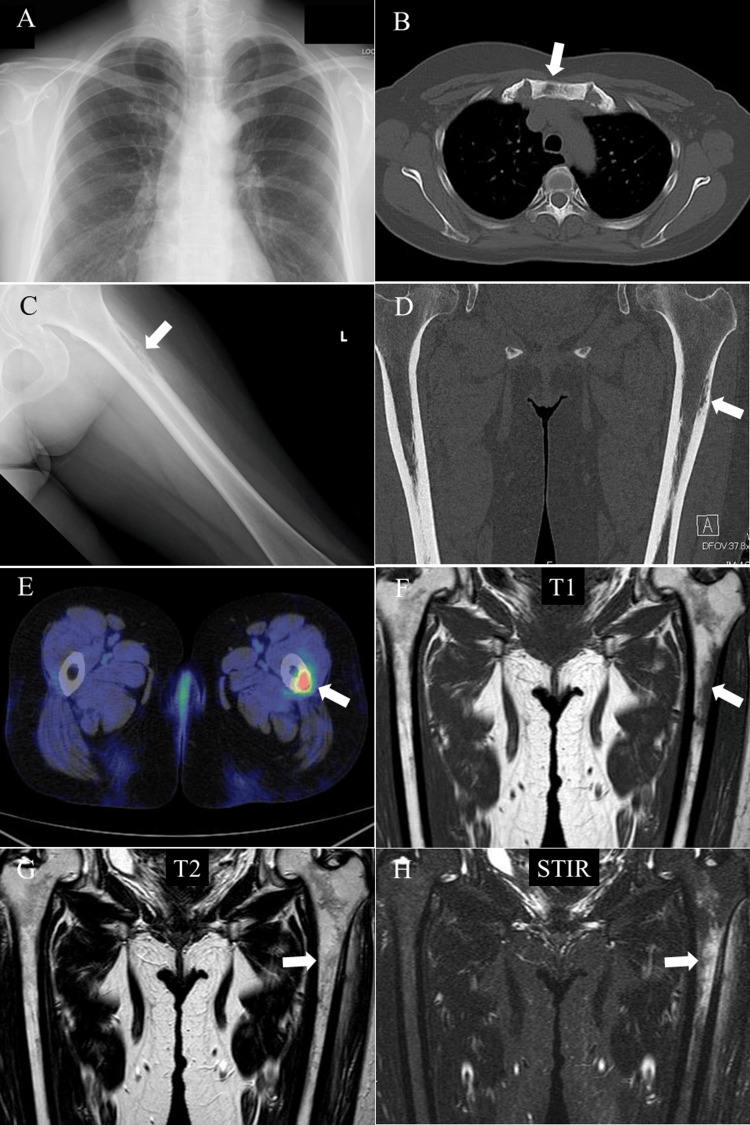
Radiological findings of pustulotic arthro-osteitis (A) Chest X-ray, (B) chest CT, (C) X-ray of the left thigh, (D) CT of the thighs, (E) positron emission tomography-CT of the thighs, (F) coronal T1-weighted MRI of the thighs, (G) coronal T2-weighted MRI of the thighs, and (H) coronal STIR-weighted MRI of the thighs. Each arrow indicates the bone lesions. CT: computed tomography; MRI: magnetic resonance image; STIR: short tau inversion recovery
